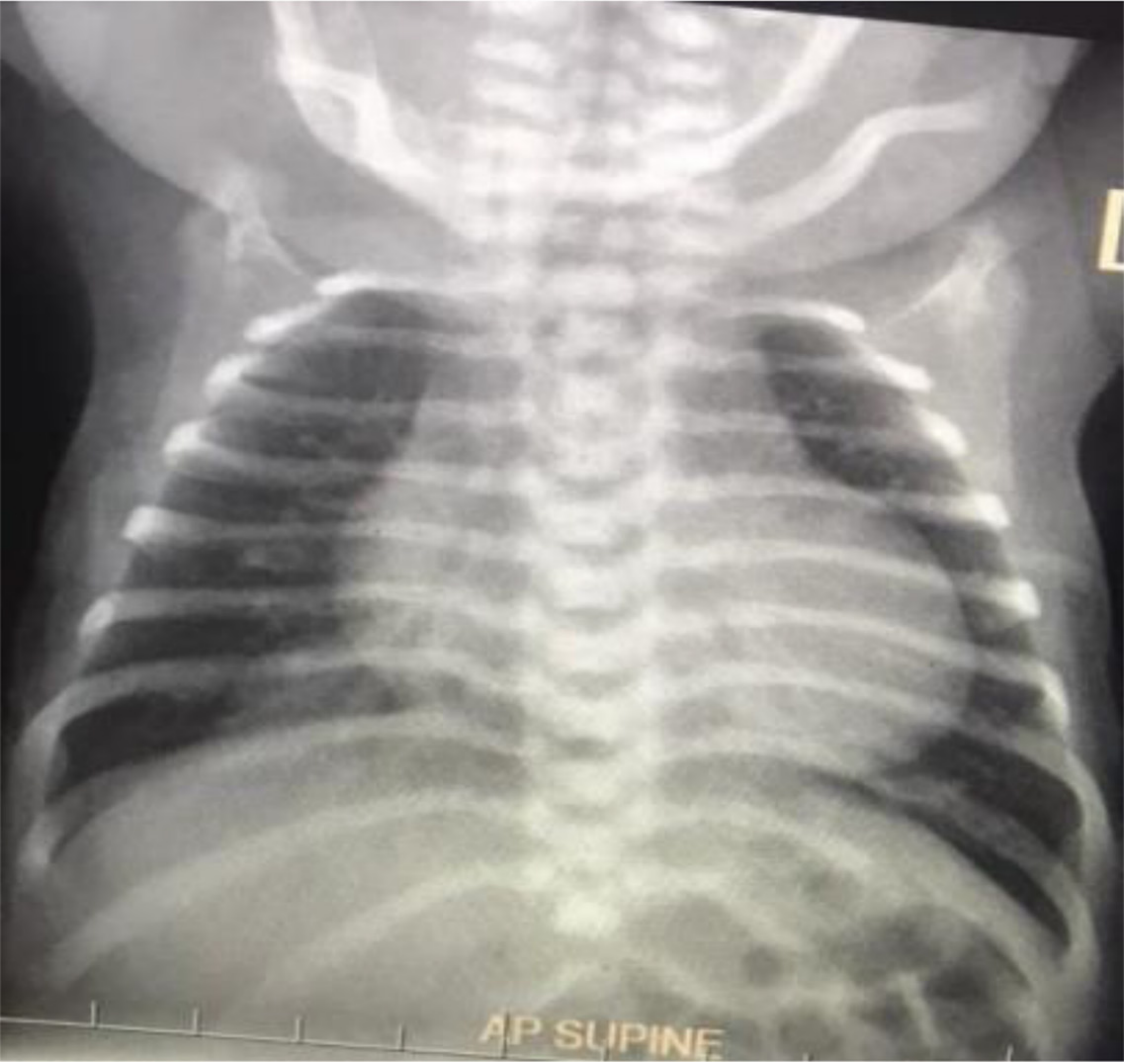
Chest X-ray showing cardiomegaly with ‘egg-on-a-string’ shape feature suggestive of transposition of great arteries.
